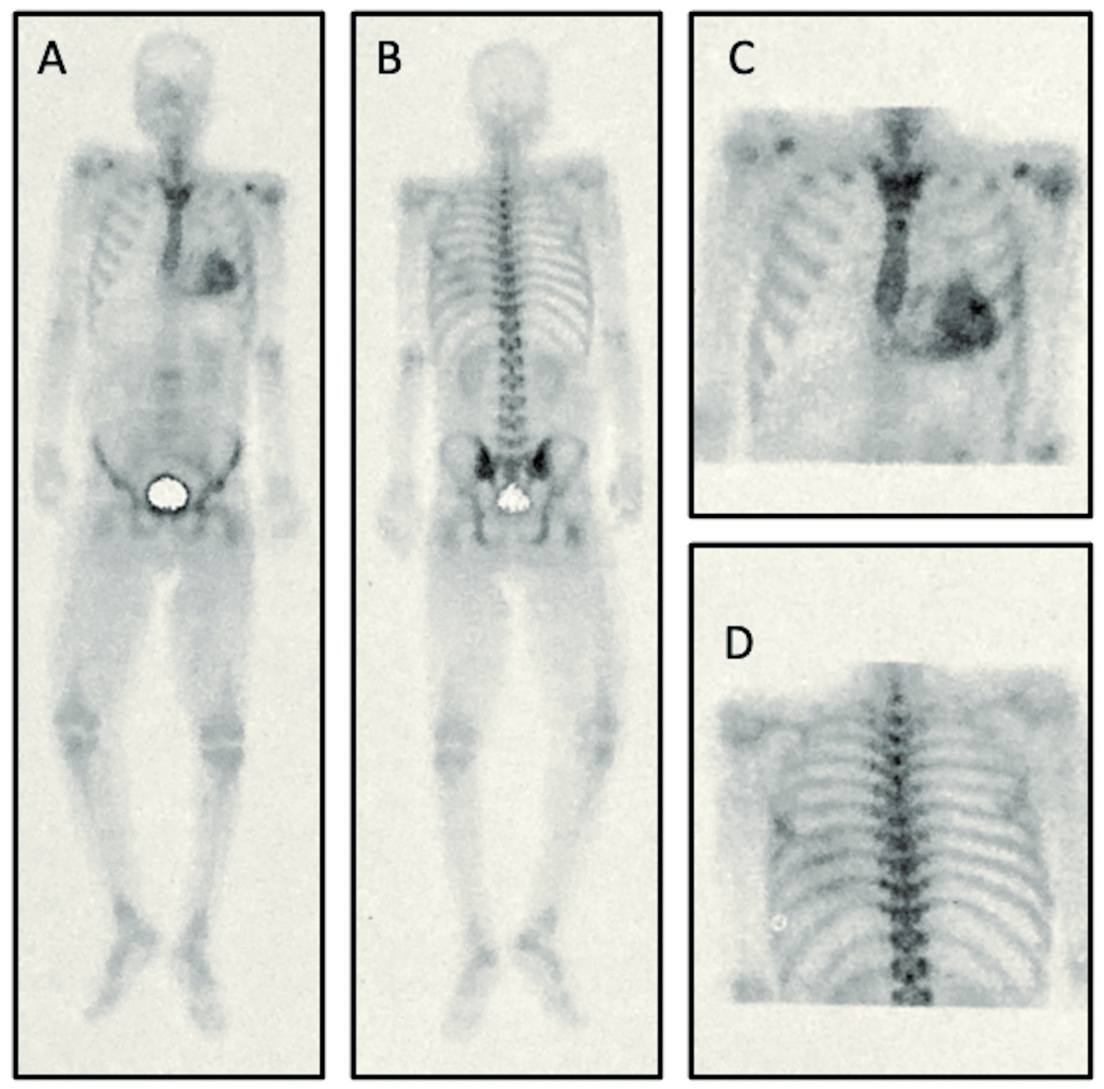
99mTc-DPD scintigraphy showing myocardial uptake suggestive of transthyretin cardiac amyloidosis (A) Whole-body planar anterior view; (B) Whole-body planar posterior view; (C) Thoracic-focused anterior view showing Perugini grade 2 myocardial uptake; (D) Thoracic-focused posterior view. In the absence of monoclonal gammopathy, this pattern is highly suggestive of transthyretin cardiac amyloidosis. 99mTc-DPD: Technetium-99m-labelled 3,3-diphosphono-1,2-propanodicarboxylic acid.
